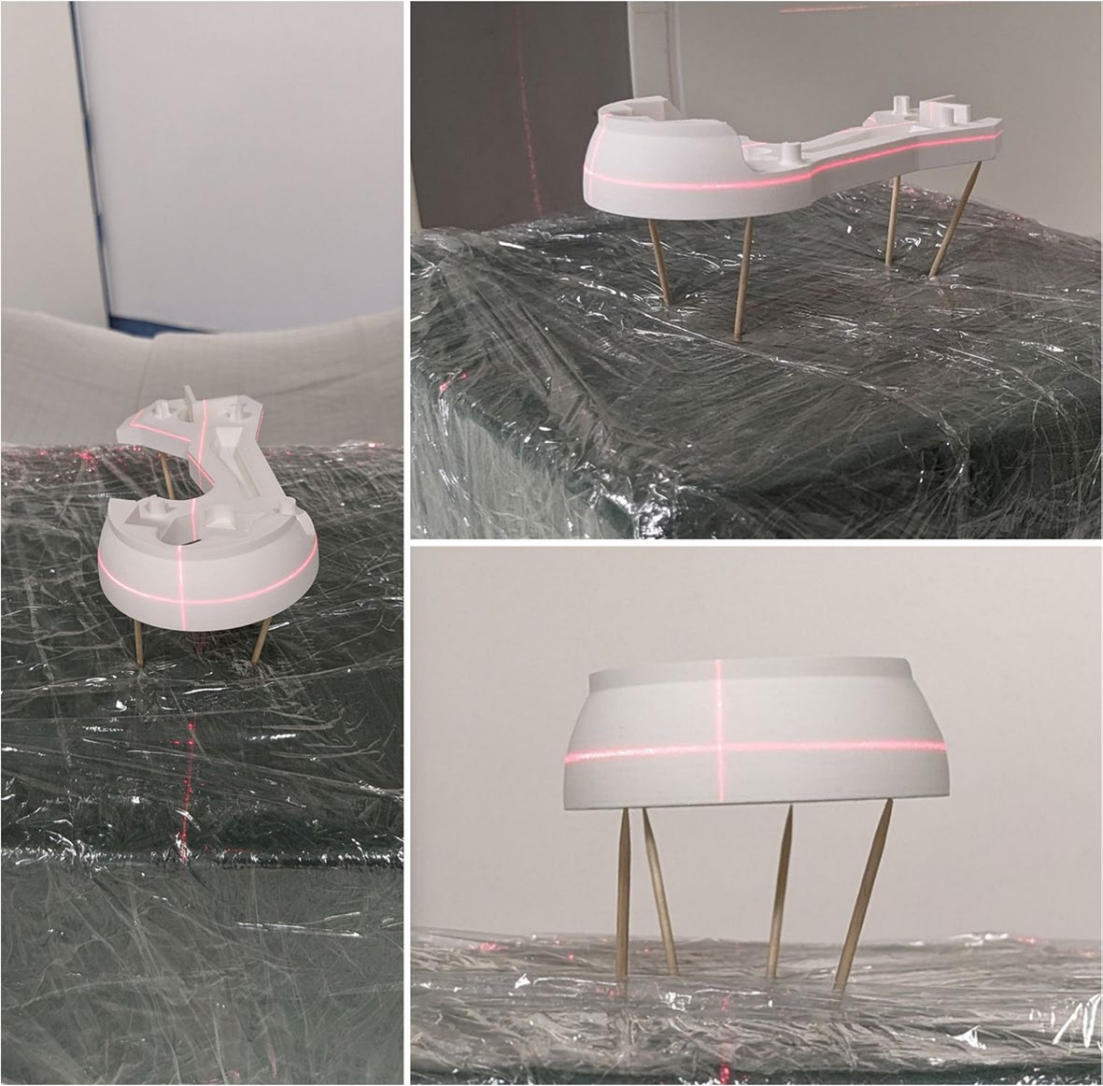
Experimental setup of the CT image acquisition
